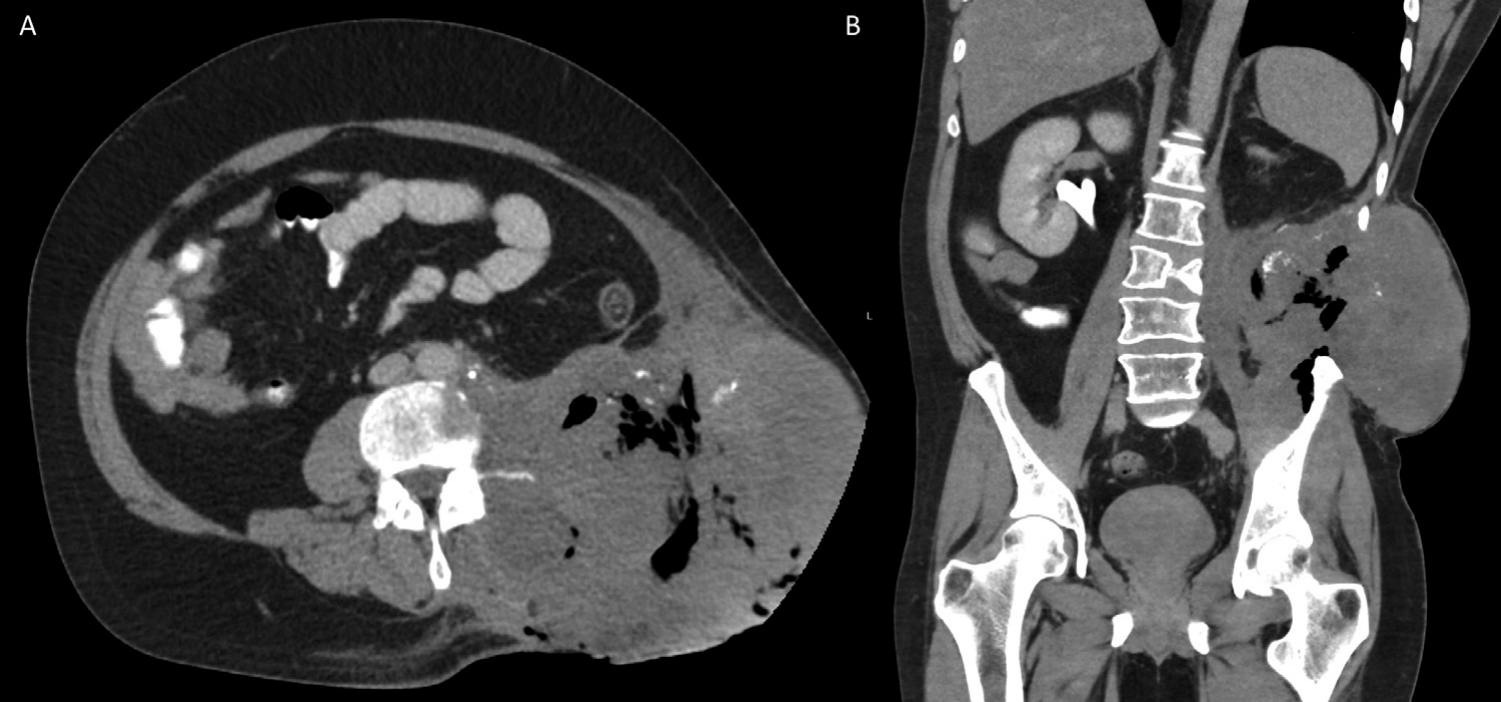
Four-years later, the patient presented with large ulcerative left flank mass (Fig. 1). Computed tomography (CT) of the abdomen and/or pelvis was performed for further evaluation, with axial (Fig. 6A), and coronal image (Fig. 6B). There was interval increase in size of the preexisting large necrotic left flank mass, which at this time had developed central necrosis with associated intramural air foci.
